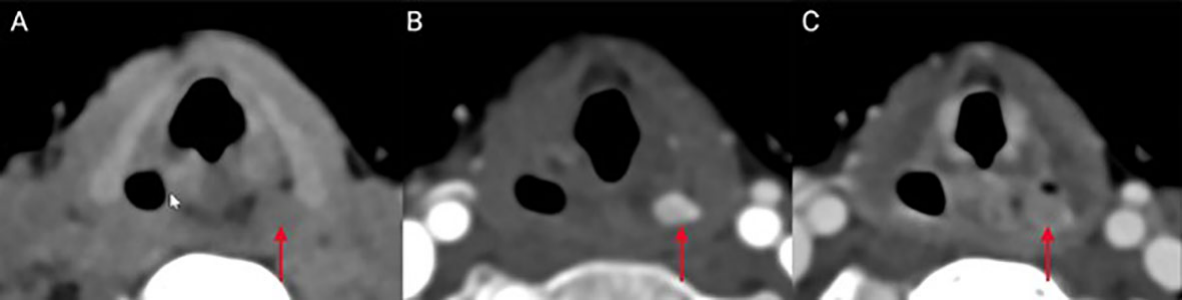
Ectopic location of the parathyroid tumor in the presented case: A left piriform sinus inconspicuous lesion (red arrow) shows similar density to adjacent muscles on CT scan images obtained prior to intravenous contrast administration (A) compared to avid enhancement on the arterial phase images (B). Delayed (venous) imaging shows fast washout of contrast from the lesion (C), consistent with diagnosis of ectopic parathyroid adenoma.
